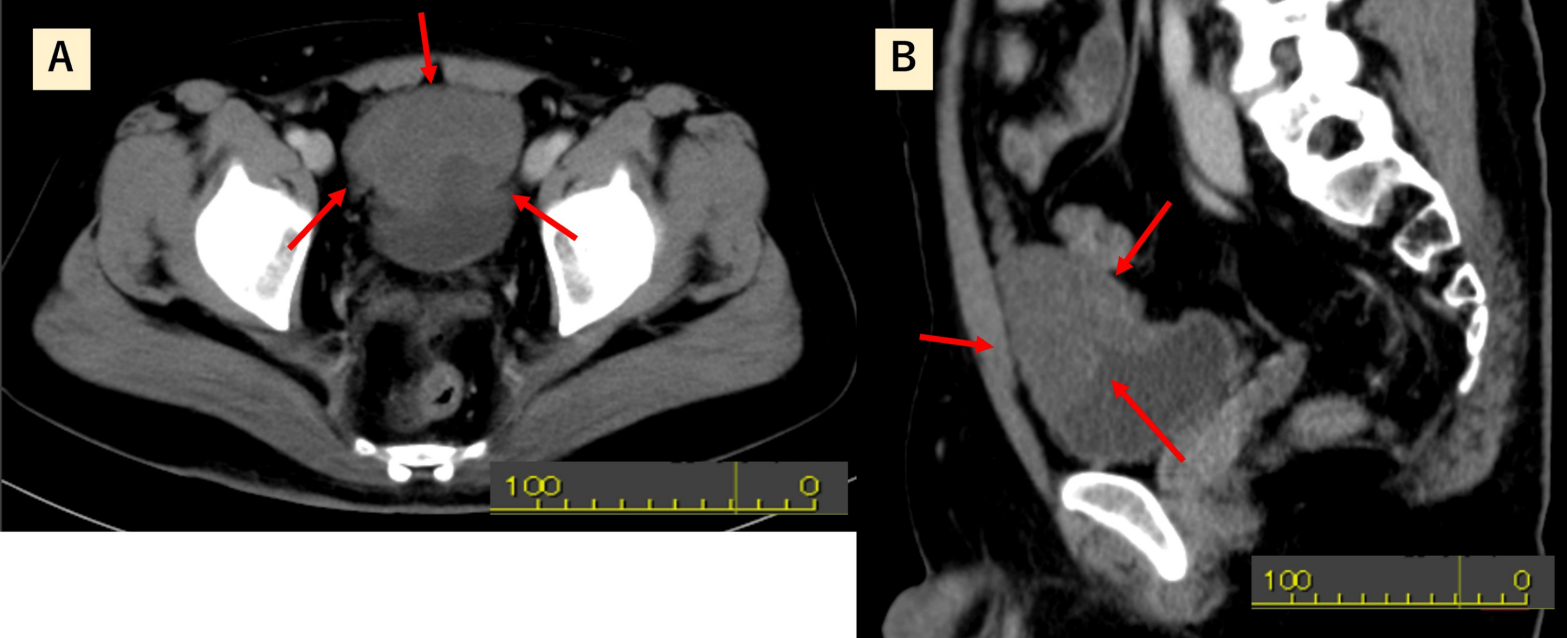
Contrast-enhanced CT images Axial (A) and sagittal (B) abdominal CT scans showing a hypoattenuating mass in the urachal region.
